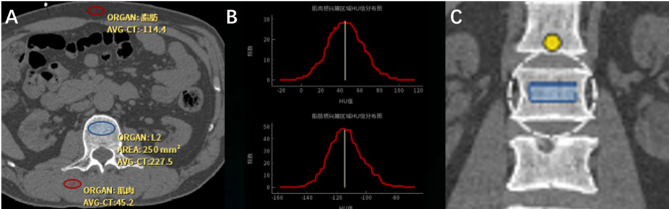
Shows the measurement of thoracolumbar vertebral BMD by using PL-QCT. The blue circle in (A) refers to the ROI of the measured vertebral body, The ROI is selected from the cancellous region in the middle of the vertebra to maximize ROI while avoiding the cortical bone, basal vein of the vertebral body, osteophyte and other areas. The red circle indicates the ROI of subcutaneous fat and paravertebral muscle on the same layer of the measured vertebral body. (B) shows that vertebral BMD is calibrated by CT values of the ROI of paravertebral muscle and subcutaneous fat on the same level of the measured vertebral body, and the ROI region needs to be adjusted to a normal distribution on the calibration curve. (C) shows coronal ROI.
